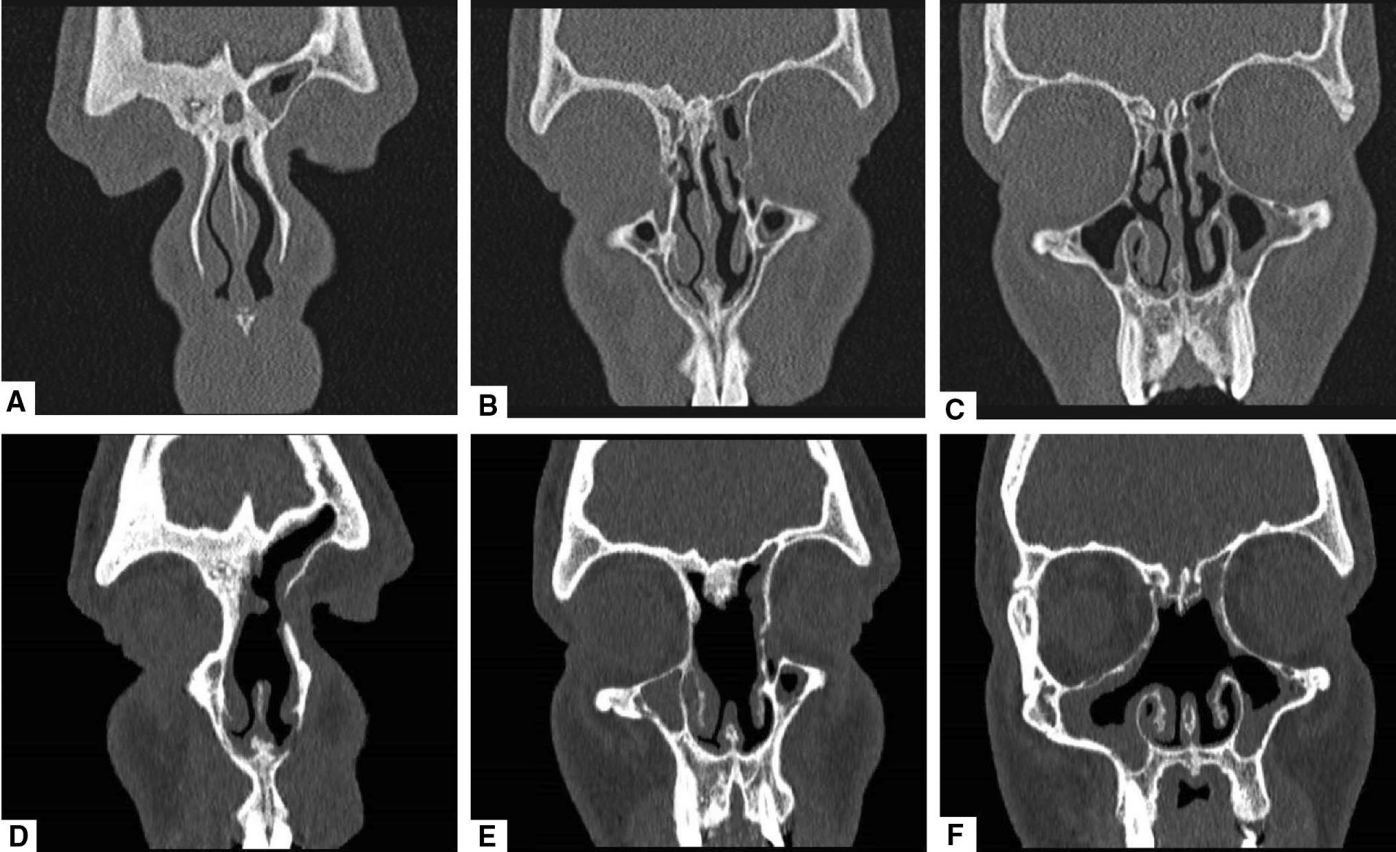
a–c Sequential slices in coronal view of the pre-operative CT scan of the 26-year-old male presenting with pott’s puffy tumour. Please note the osteitis of the frontal bone blocking the frontonasal pathway as a result of the previous endoscopic sinus surgeries and the ongoing inflammation. d–f Sequential slices in coronal view of the post-operative CT scan 13 months after Draf III
